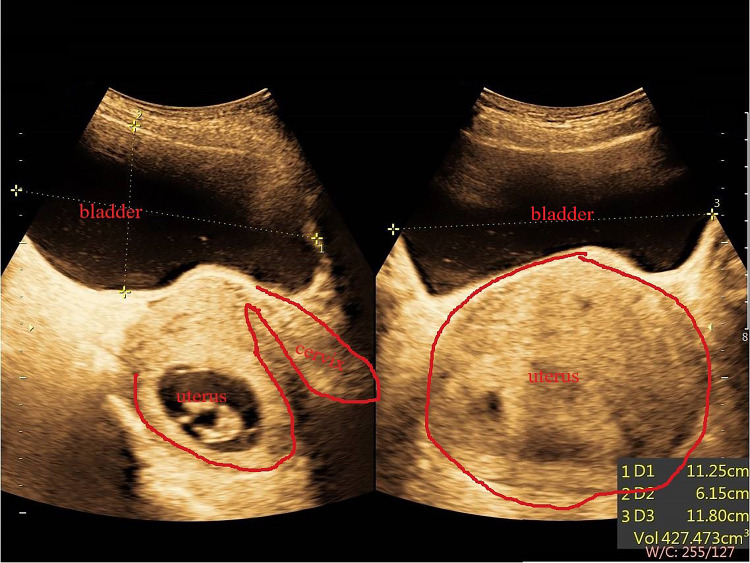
Abdominal ultrasound shows a retroverted uterus and a full bladder. Abdominal ultrasound shows a retroverted uterus and a full bladder. The left image is the longitudinal section of the probe, and the right image is the transverse section of the probe.
